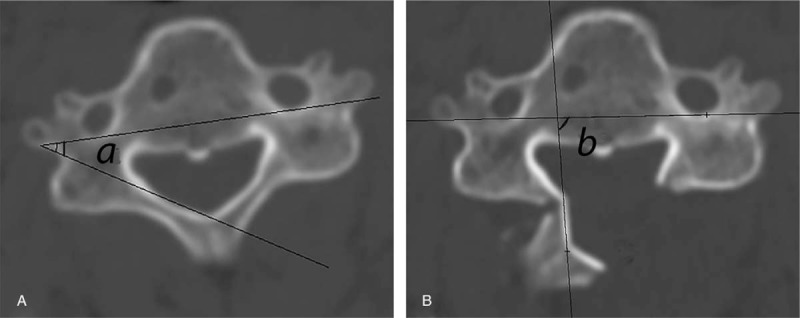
The different laminae angles were measured on computed tomography films. (A) Preoperative lamina angle a. (B) Postoperative lamina angle b. The lamina open angle = Postoperative lamina angle b − Preoperative lamina angle a.
